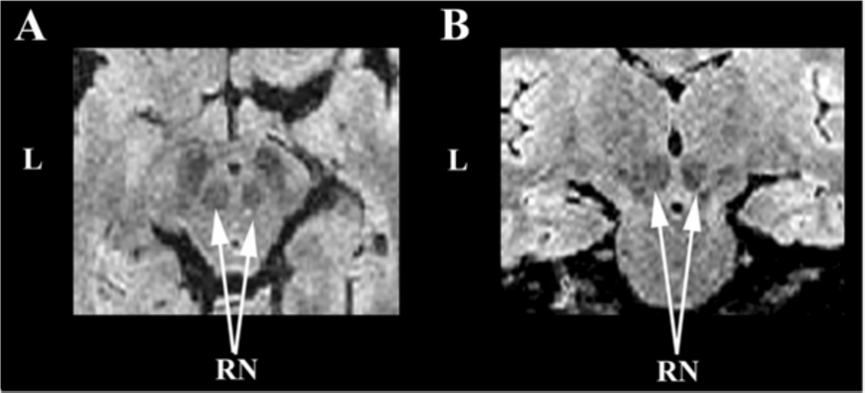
FLAIR-CUBE image of the red nucleus (RN) whithin the mesencephalon showing a reduced volume of the right nucleus compared to the left one. A. Axial slice. B. Coronal slice. L. Left side.
